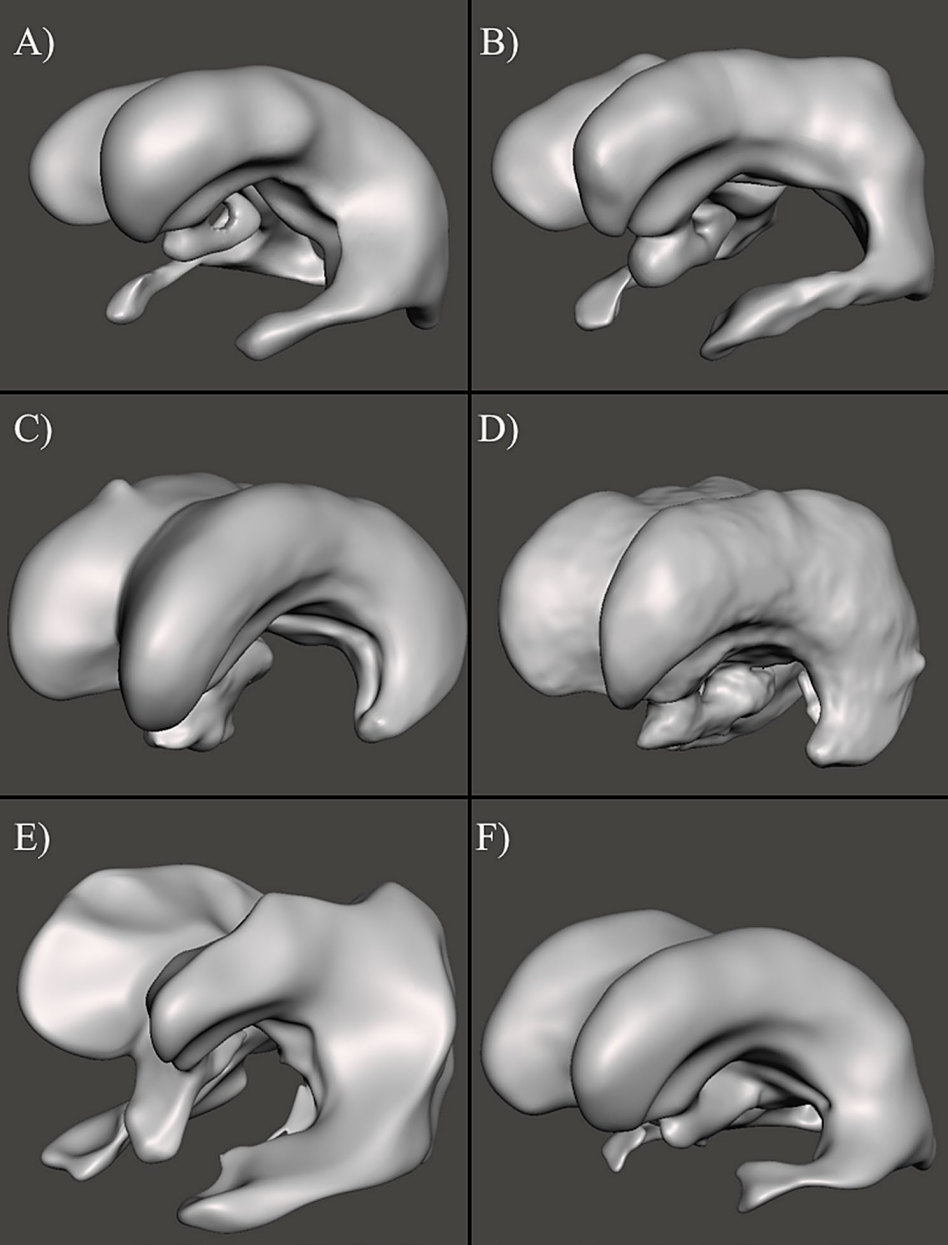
Front-left views of 3D ventricle models derived from patient MRI data. 3D models were loaded into Autodesk Meshmixer for imaging. Additional views of each model (lateral, cranial, caudal) are available in Supplementary Figs. 1–6. (A) *P-0* MRI model (B) *P-1* MRI model (C) *P-2* MRI model (D) *P-3* MRI model (E) *P-4* MRI model (F) *P-5 (II)* MRI model
